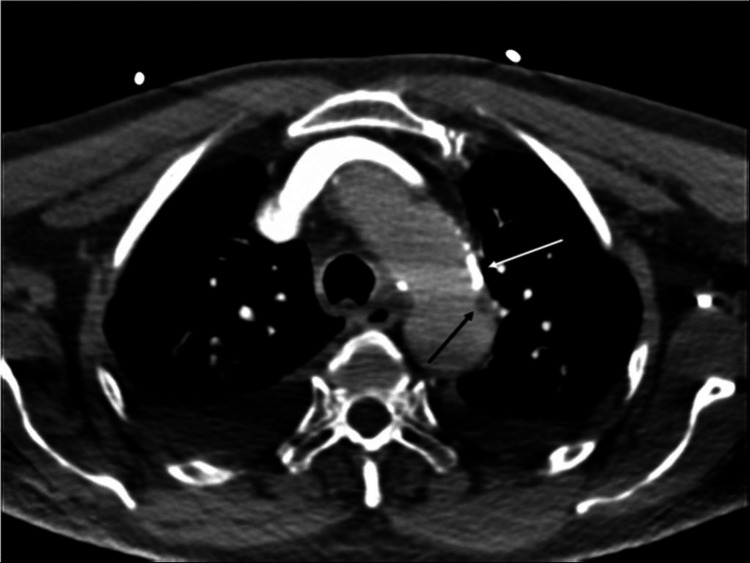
Abdominal computed tomography, transverse section showing the aorta A transverse section of an abdominal computed tomography with contrast in which the white arrow represents calcified atherosclerotic changes while the black arrow represents soft atheroma or noncalcified changes in the aortic arch
